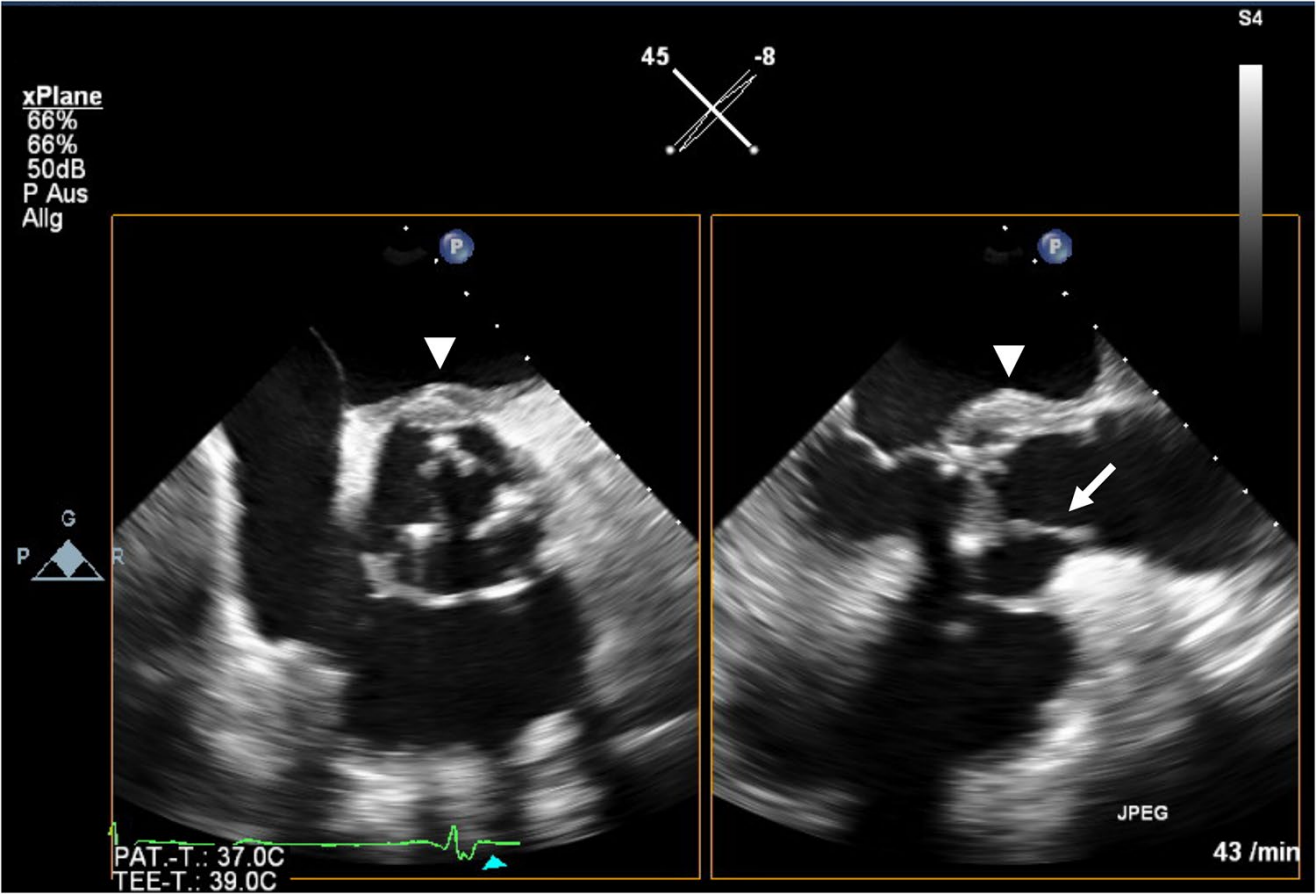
Transesophageal echocardiography shows a hyperechoic mobile mass attached to the aortic valve (arrow) and perivalvular swelling (arrowhead) consistent with infectious endocarditis and concomitant abscess formation
